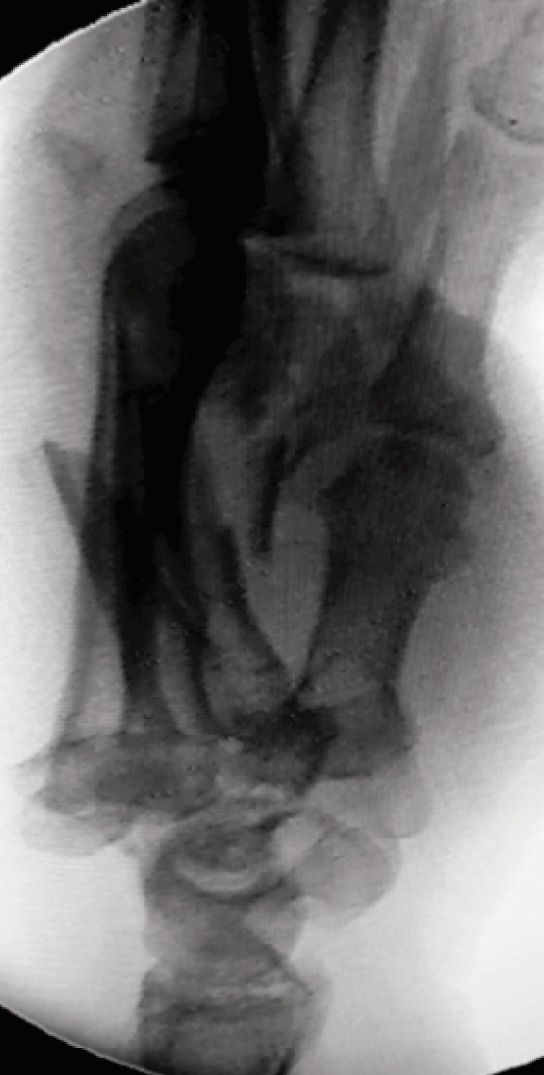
Lateral radiograph view showing Classification Type C.1. Divergent dislocations of multiple metacarpals with concomitant metacarpal shaft fractures.
